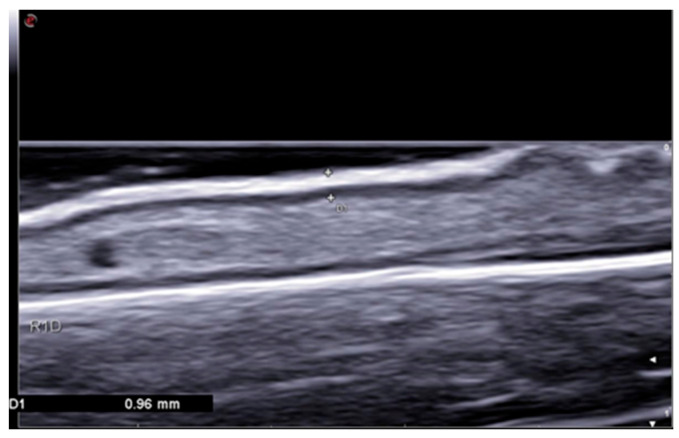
Ultrasonography longitudinal scan at the level of the third phalanx showing a skin thickness measurement that includes epidermal and dermal layers.
